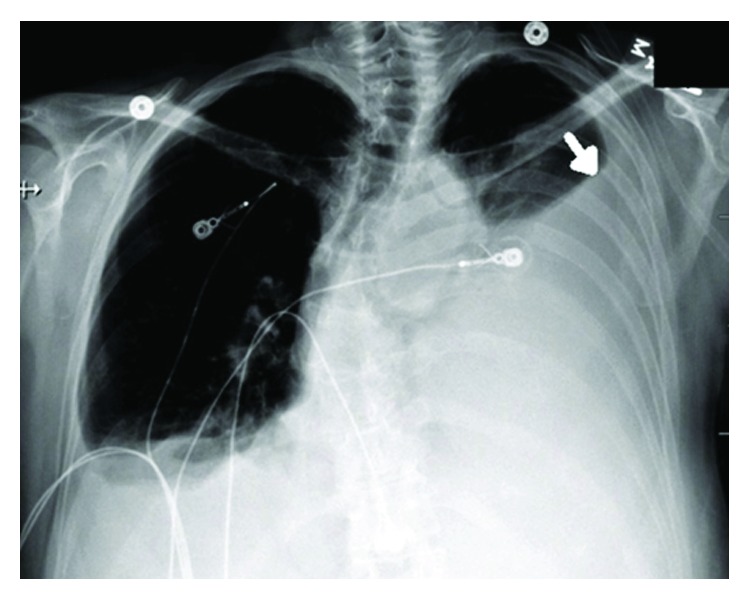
PA chest radiograph showing a large left-sided pleural effusion (arrow).
